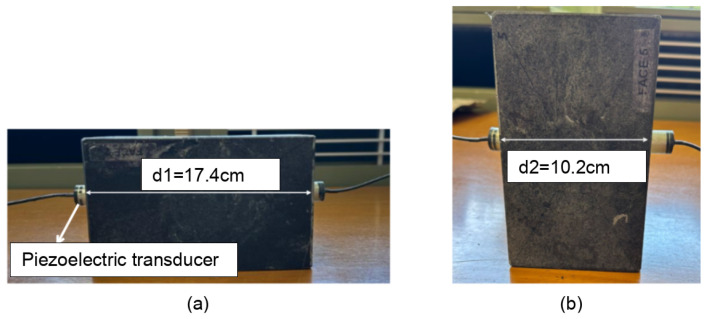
A reference material, namely marble, was used to calibrate the piezoelectric transducers. By measuring ultrasonic wave velocity at two different propagation distances d1 in (a) and d2 in (b) we can estimate the propagation time of the wave within the transducers. Note: The two depicted transducers are identical in function, although their external appearance differs slightly due to variations in the application of waterproof coating for technical purposes.
